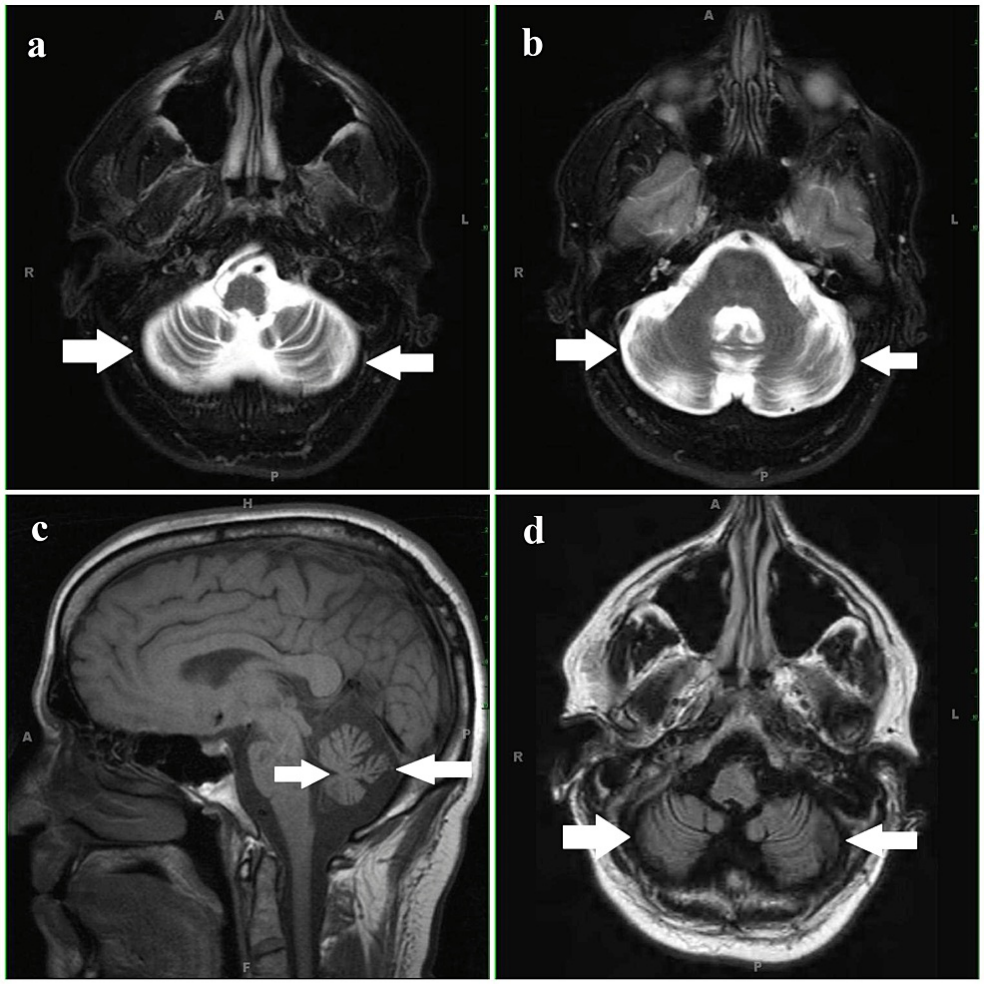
MRI of the brain a, b: MRI of the brain (T1) axial section with contrast showing cerebellar degeneration with the prominence of adjacent subarachnoid space. c: MRI of the brain (T1) sagittal section showing cerebellar degeneration. d: MRI of the rain (T1) axial section without contrast showing cerebellar degeneration with the prominence of adjacent subarachnoid space.
